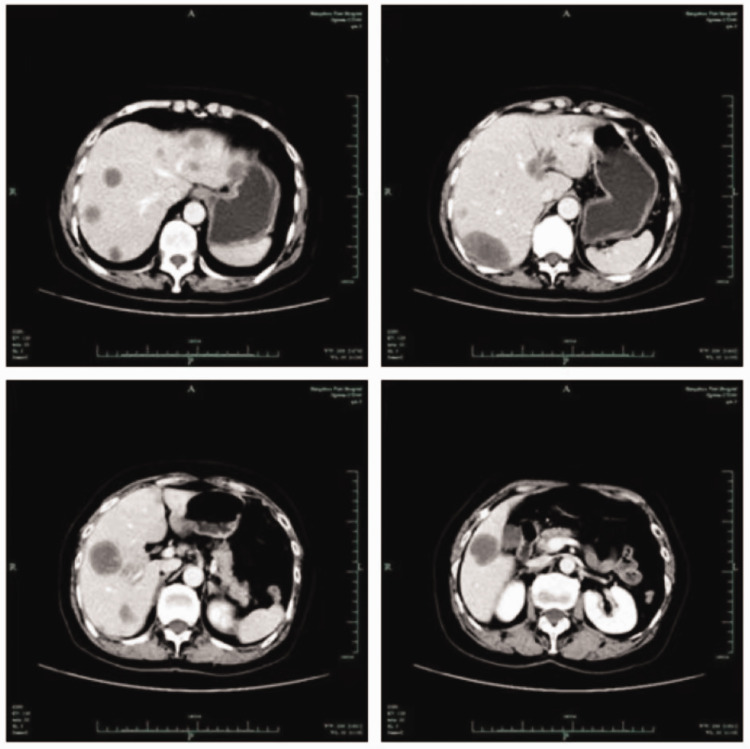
Enhanced computed tomography showing multiple liver space-occupying lesions.
